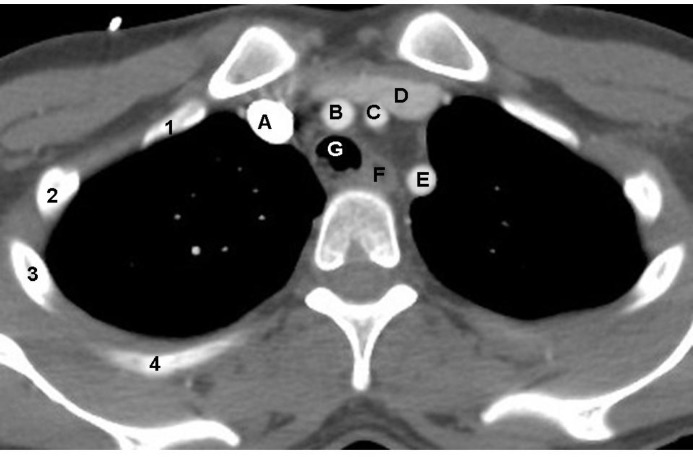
On contrast enhanced axial CT image of the chest of a 41 years old man shown above, identify the labelled normal anatomical structures labelled A to G.
